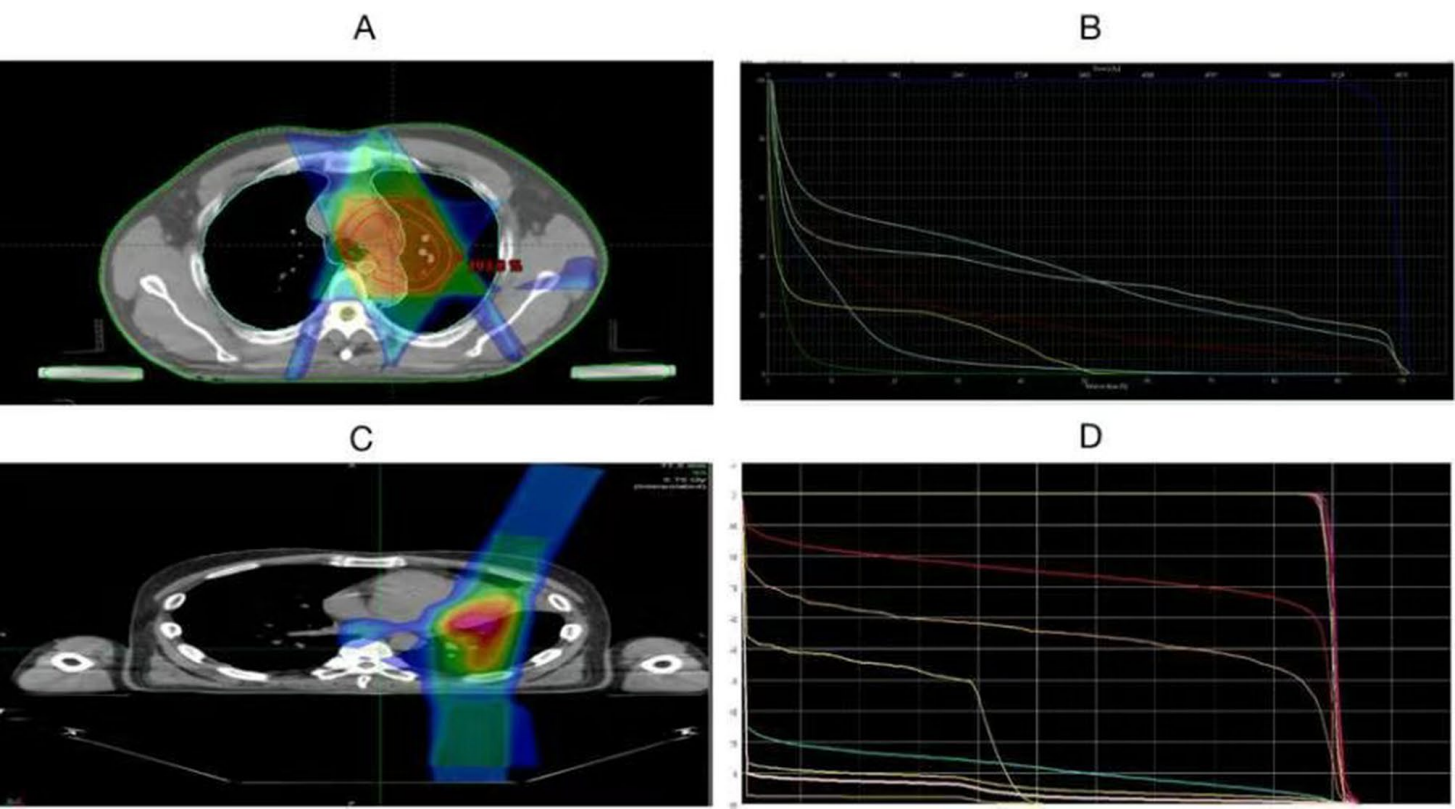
Dose Distribution and DVH. Note: A and B represent the dose distribution and DVH (Dose-Volume Histogram) for intensity-modulated radiation therapy (IMRT) with X-rays, respectively; C and D represent the dose distribution and DVH for fixed-angle proton therapy, respectively
